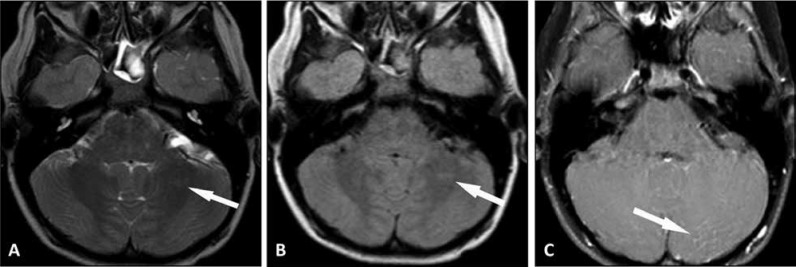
Initial brain MRI exam. (A) Axial T2-weighted image, (B) axial FLAIR image showing subtle 10 x 4 mm focus of T2 prolongation in the white matter of the left cerebellar hemisphere and (C) mild diffuse leptomeningeal enhancement was present overlying the left posterior cerebellar hemisphere.
